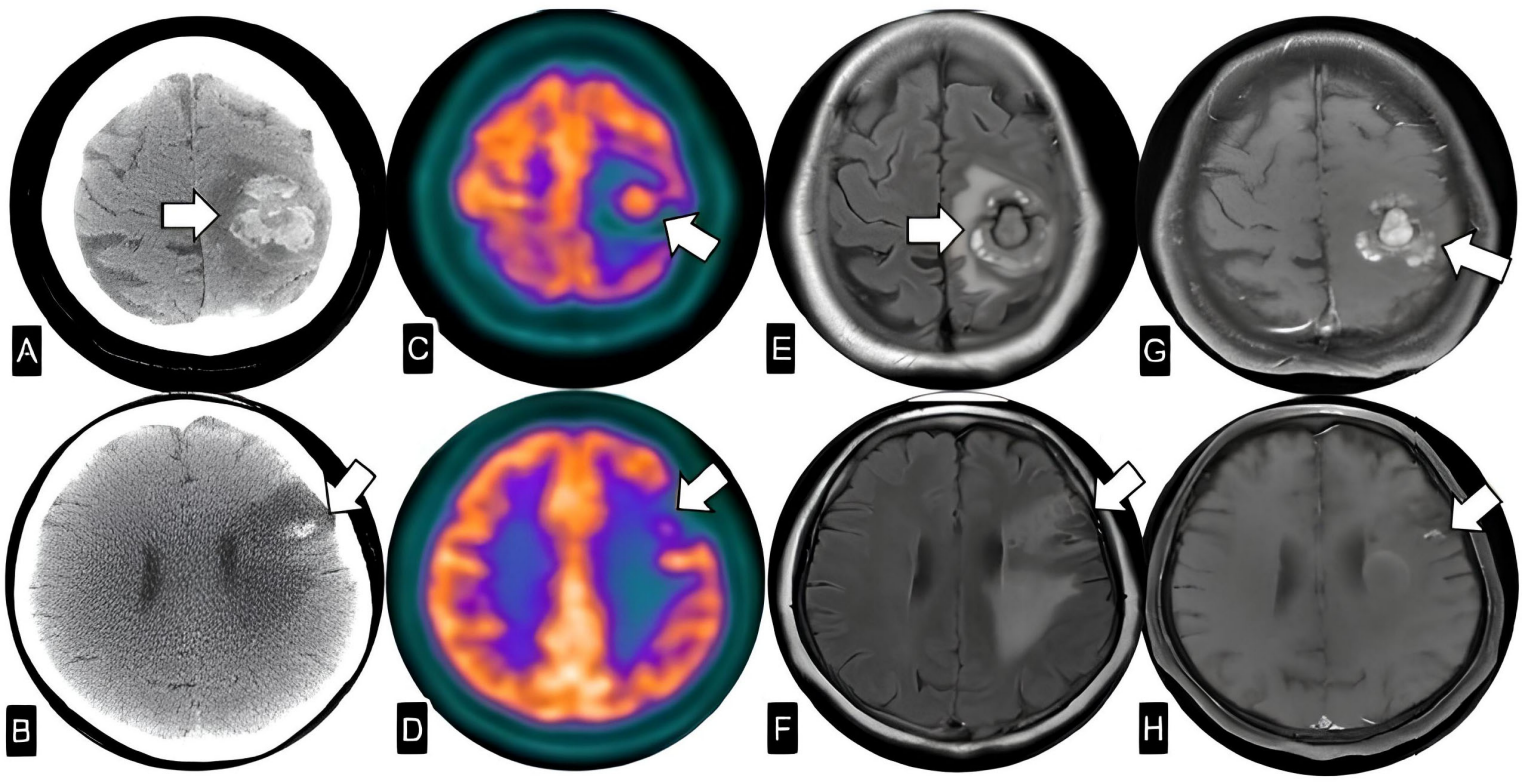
(A, B) Non-enhanced CT images. (C, D) Fused 18FDG-PET andCT images. (E, F) T2 FLAIR and (G, H) T1-Gd axial MRI. The images reveal two intraparenchymal expansive hemorrhagic and enhancing lesions in the left frontoparietal region with FDG uptake, suggestive of metastatic disease.
